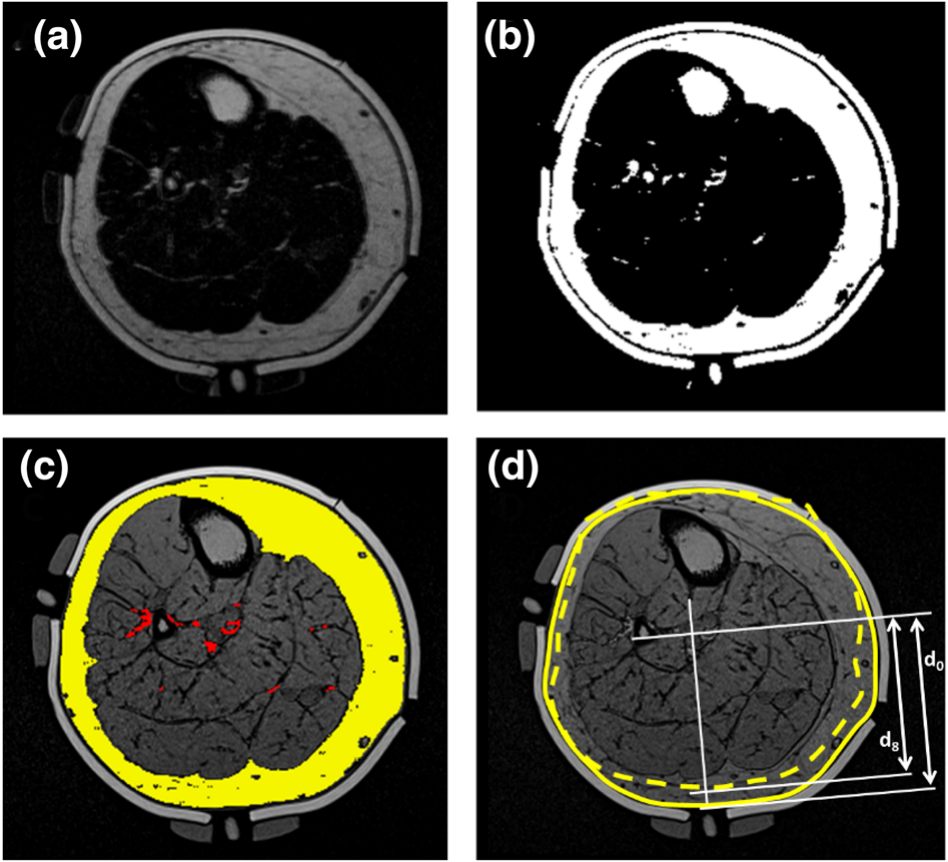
Image processing steps applied to the axial MRI fat-only slice of the lower limb at the posterior calf measurement site, showing (a) original image, and (b) after binarization and masking. (c) superficial adipose mask (yellow) and muscle-infiltrating adipose mask (red) superimposed over the corresponding opposed-phase image at same slice, and (d) superimposed outlines of limb under uninflated cuff baseline (solid line) and 8 kPa inflated cuff (dashed line) conditions. Example measures are shown for calculating the displacement and gross strain arising from cuff inflation between the posterior calf indenter to the nearest bony prominence, uninflated (*d*_*0*_) and at 8 kPa inflation (*d*_*8*_).
